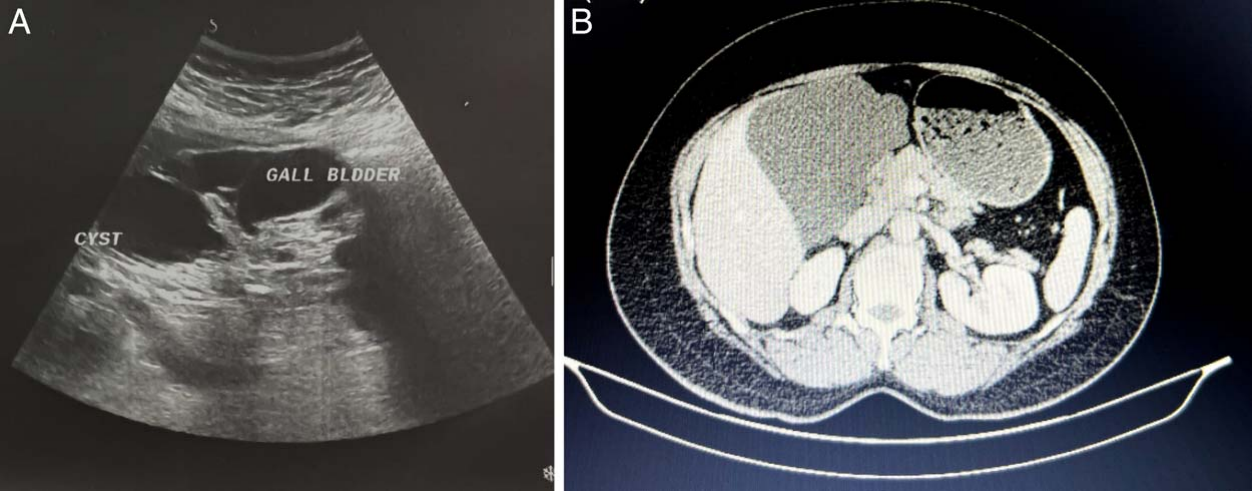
(A) A large multilobulated cystic lesion in ultrasonography is shown. (B) Abdominal computed tomography demonstrates a large lobulated non-enhancing wall cystic lesion in gallbladder fossa.
